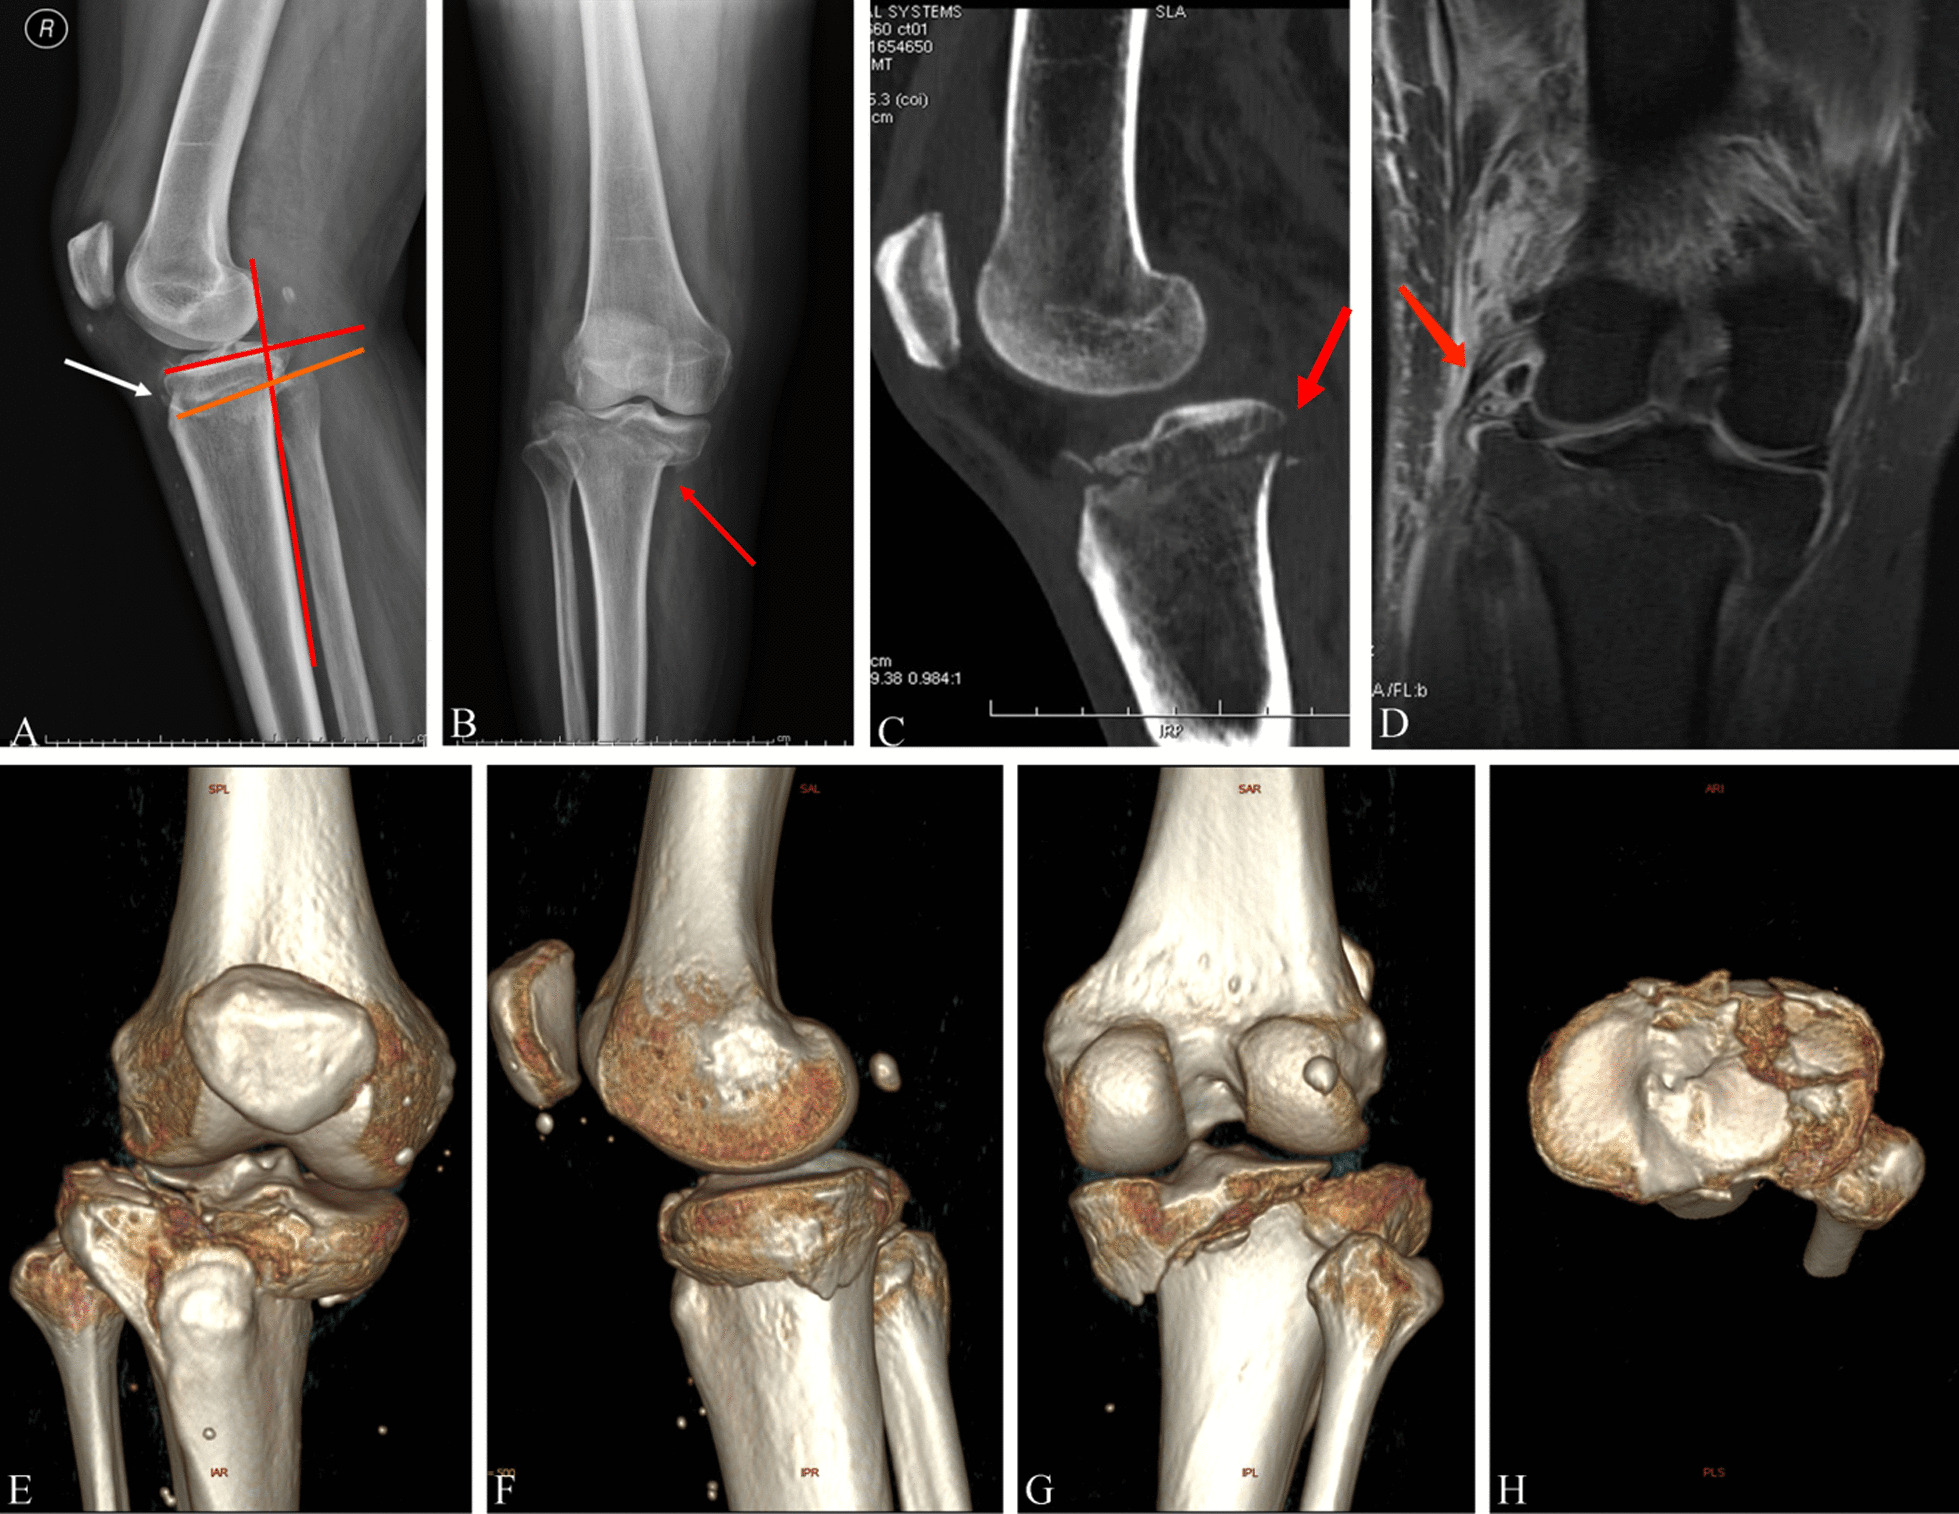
Hyperextension varus bicondylar fracture due to a fall from height. Radiological images of a 59-year-old Chinese male with HBTPF. a Red line and orange line showed the reversed slope. White arrow shows the small bone fragment of the fracture. b The red arrow showed the anterior compression fracture. c Red arrow showed the tension failure. d–h 3D computed tomography reconstruction preoperatively
